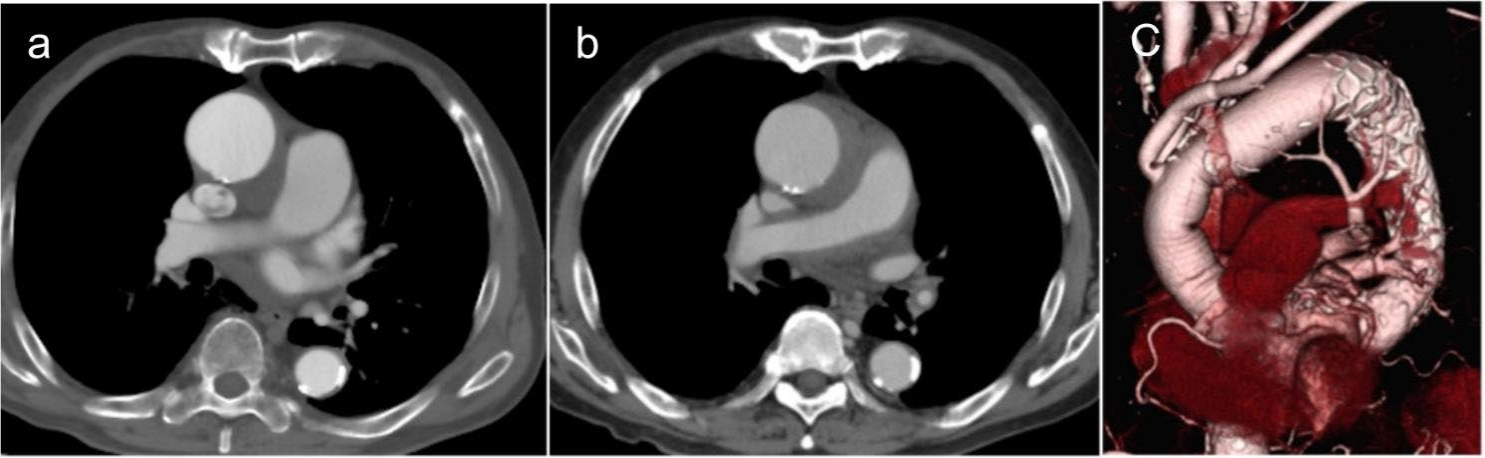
Acute type A aortic dissection with a non-communicating false lumen 3 months before surgery (a). Two day before surgery (b). Preoperative computed tomography image (c)
